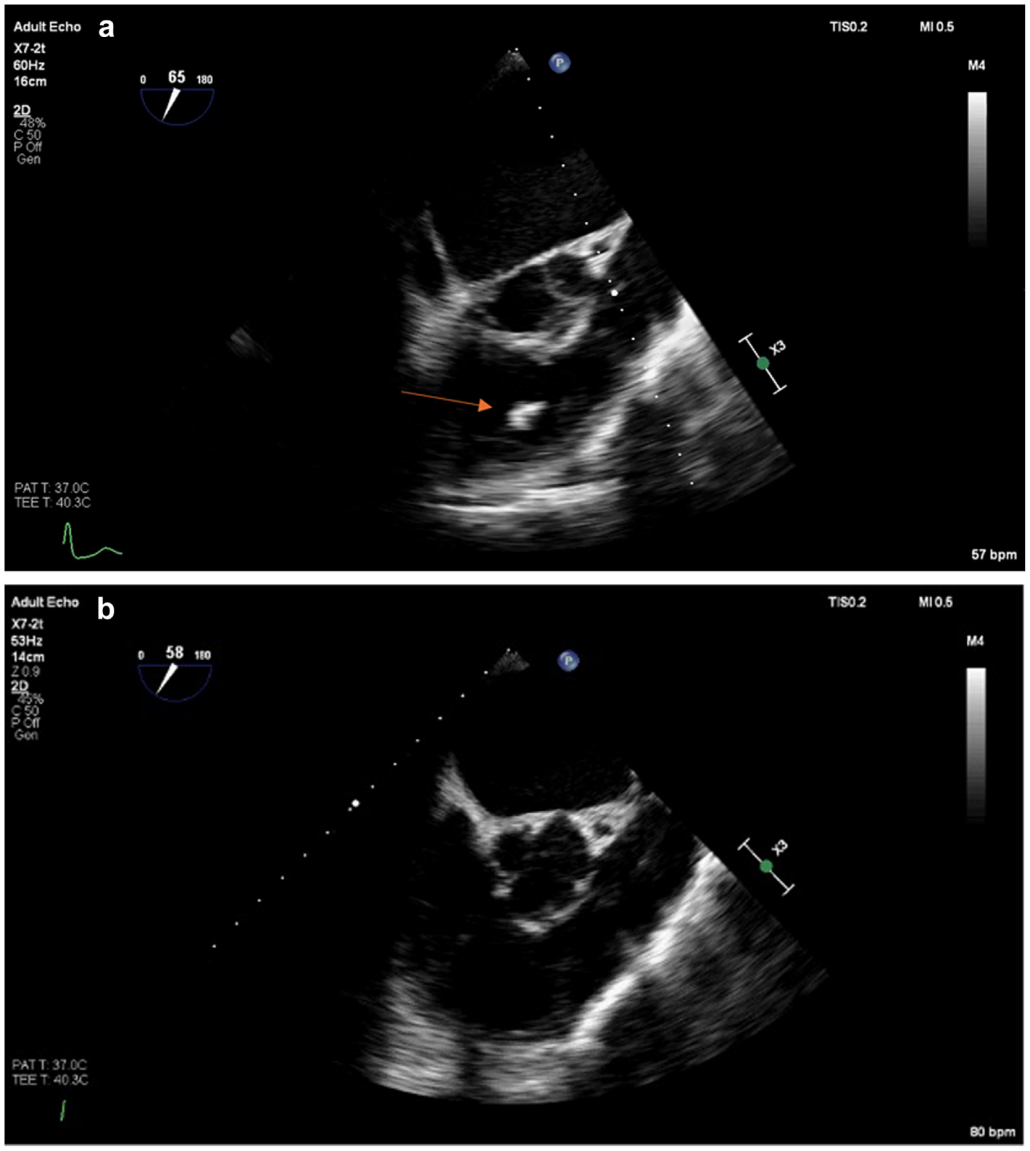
A, Clot-in-transit (CIT) within the right atrium as demonstrated on transesophageal echocardiogram (TEE) and marked with an *orange arrow*. B, TEE performed 1 day after the suction thrombectomy demonstrating that the CIT is no longer present in the right atrium or right ventricle (RV).
